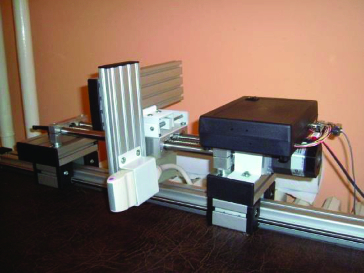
Positioning mechanism of the ultrasound probe.
